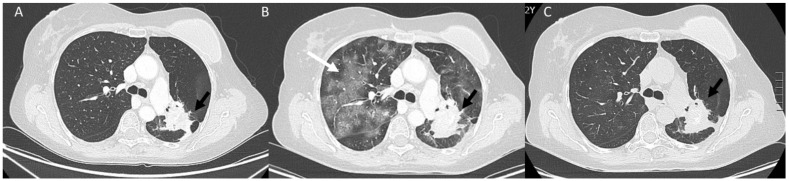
NSLC (black arrows) patient treated with immunotherapy. CT assessment in pre-treatment phase (A). During follow-up, in (B), appearance of ir-Pneumonitis (white arrow), with disease regression after corticosteroid treatment (C).
